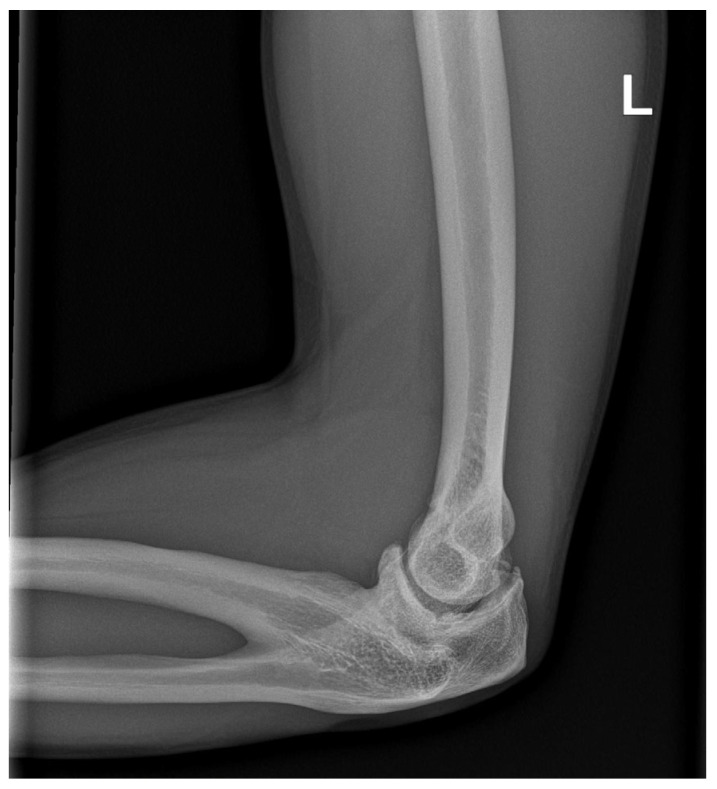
Radiographic image of the elbow joint in lateral view. Markable osteophytic changes of olecranon and coronoid process are present.
